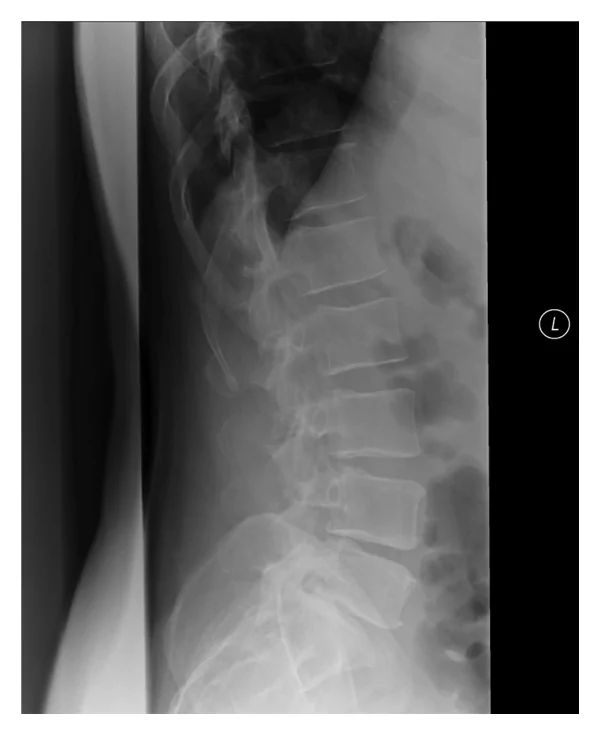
Lumbar spine radiographs demonstrate disc space narrowing at L4–L5 and L5–S1, with no evidence of fracture, spondylolysis, or spondylolisthesis.
